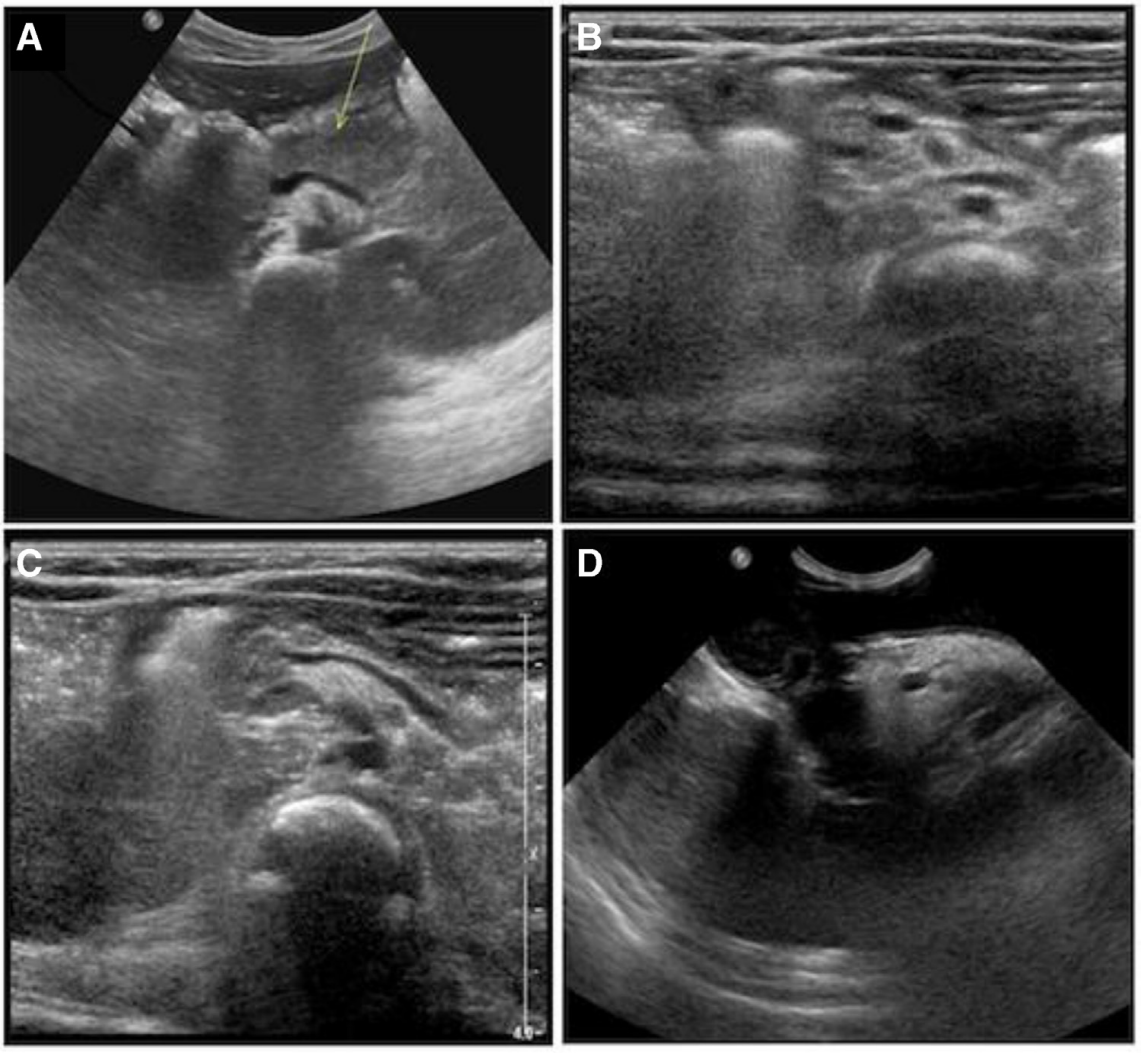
US images. (A) Normal pancreas size and echogenicity of a healthy 2-month-old infant. (B, C) Reduced thickness and abnormal echogenicity of the pancreas at 2 months of age in the present case. (D) Hyperechoic pancreas with increased size compared with the previous US appearance at 9 months of age. US = ultrasound.
